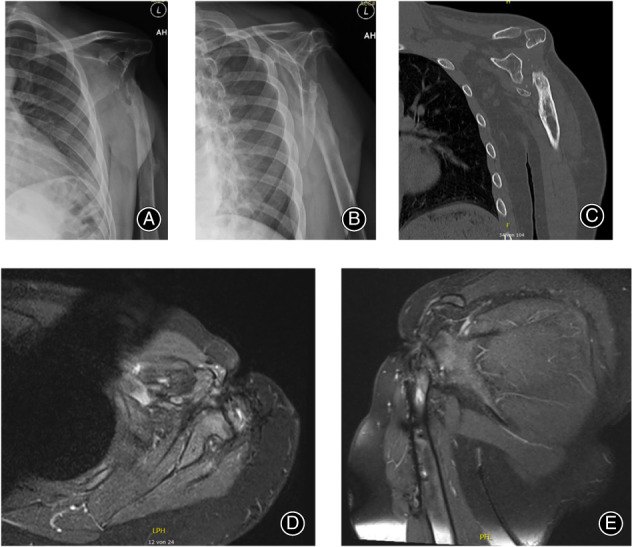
(A) and (B) Preoperative X‐ray in two planes with proof of progressive destruction of the glenohumeral joint. (C) Coronal slice of the CT. (D) and (E) Axial and coronal MRI with proof of insufficient rotator cuff and advanced soft tissue destruction
